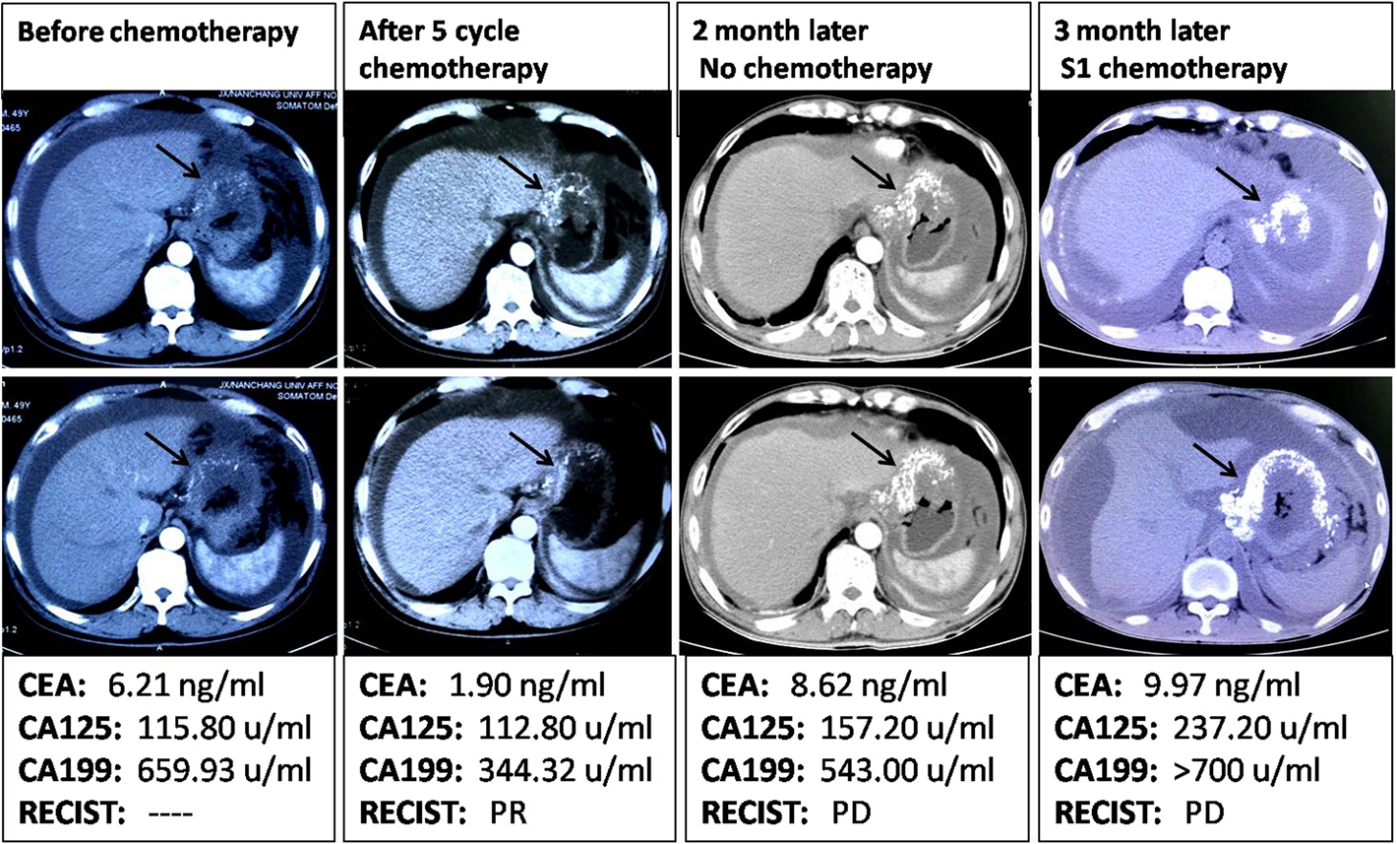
Serial abdominal CT scans show continuous increase in the calcifications (arrow) since starting chemotherapy
